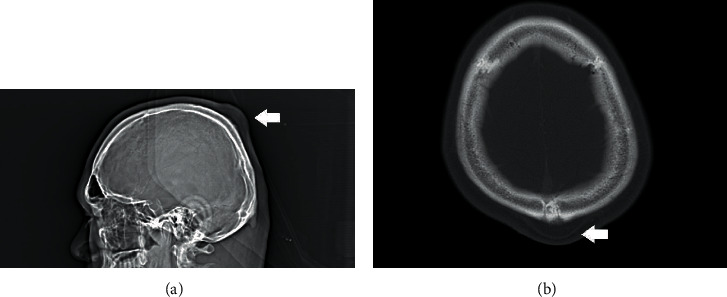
Computed tomography of the cranium showing a posterior scalp mass at the midline near the convexity: (a) sagittal view; (b) transverse view: 3.7 cm at the greatest dimension.
